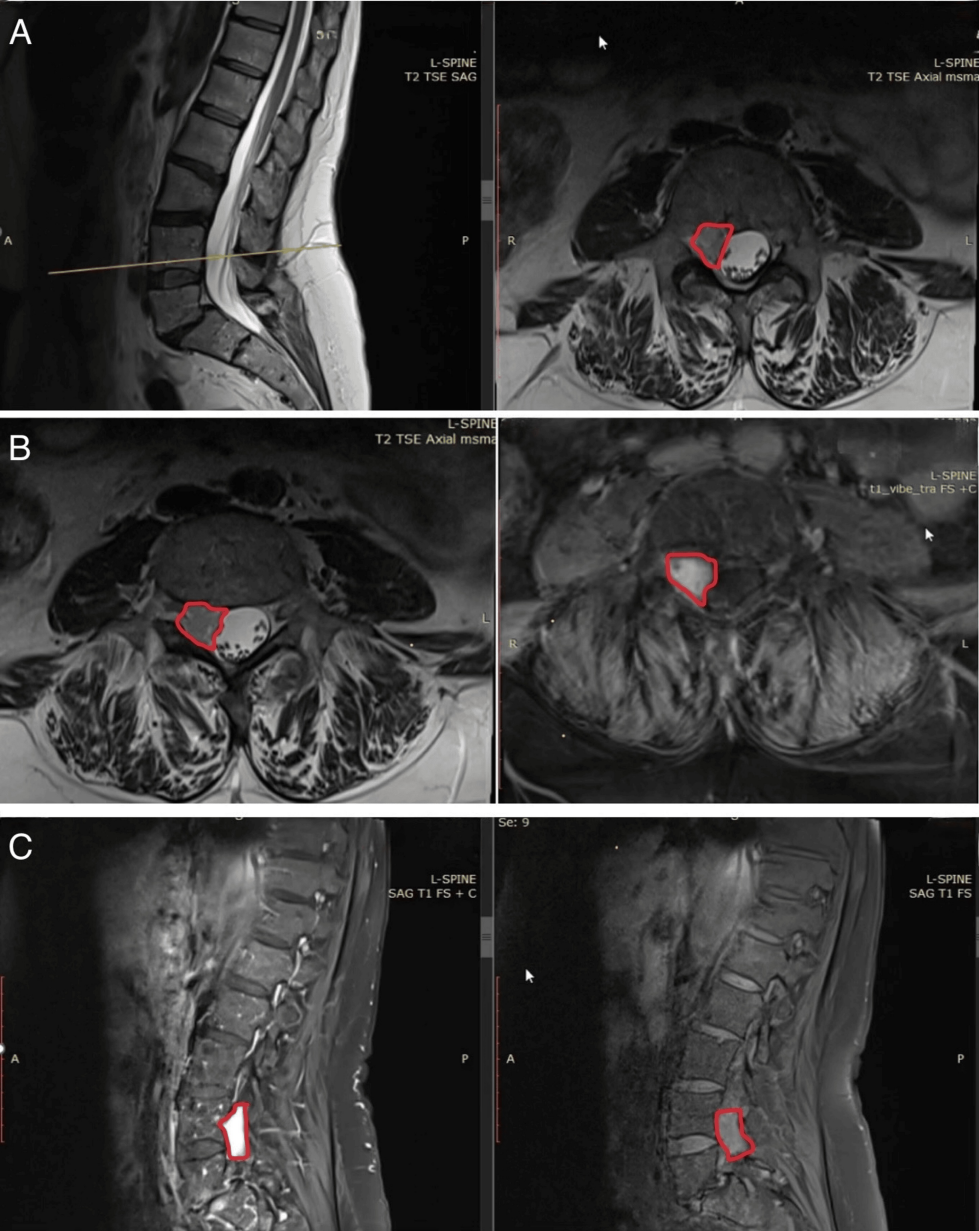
Magnetic resonance imaging of the lumbar spine showing an extradural lesion at the L4-L5 level (A) Sagittal (left) and axial (right) T2-weighted images demonstrating a well-circumscribed extradural lesion (outlined in red) located posterior to the L4 vertebral body, causing slight compression of the dural sac and the contained nerve roots. (B) Axial T2-weighted (left) and T1-weighted postcontrast (right) sequences showing the same lesion with intermediate signal intensity on T2 and intense enhancement after gadolinium injection, consistent with an extradural mass. (C) Sagittal T1-weighted (right) and T1-weighted postcontrast (left) images confirming homogeneous contrast enhancement of the lesion, better delineating its extradural location and its relationship with surrounding structures
